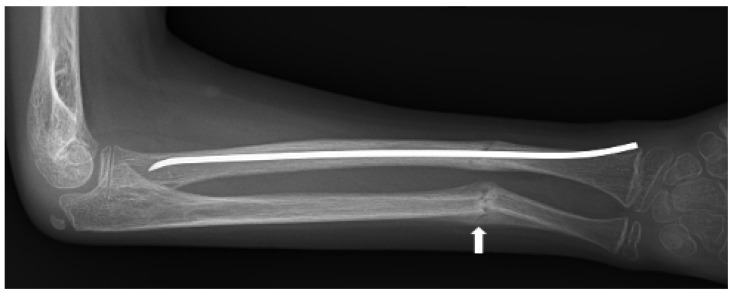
Anteroposterior radiograph of right radial and ulnar fractures of a patient with *LRP5* variant-induced familial exudative vitreoretinopathy (FEVR). The fractures, which were initially undisplaced (Figure 3), became angulated, encroaching upon the interosseous space and potentially limiting supination and pronation. Preservation of rotational motion of the forearm was achieved by intramedullary rodding of the radius, restoring physiological alignment. Fracture callus is seen at the ulnar fracture (white arrow). Due to the young age of the patient, the residual angulation of the ulna underwent remodeling to a physiological position.
